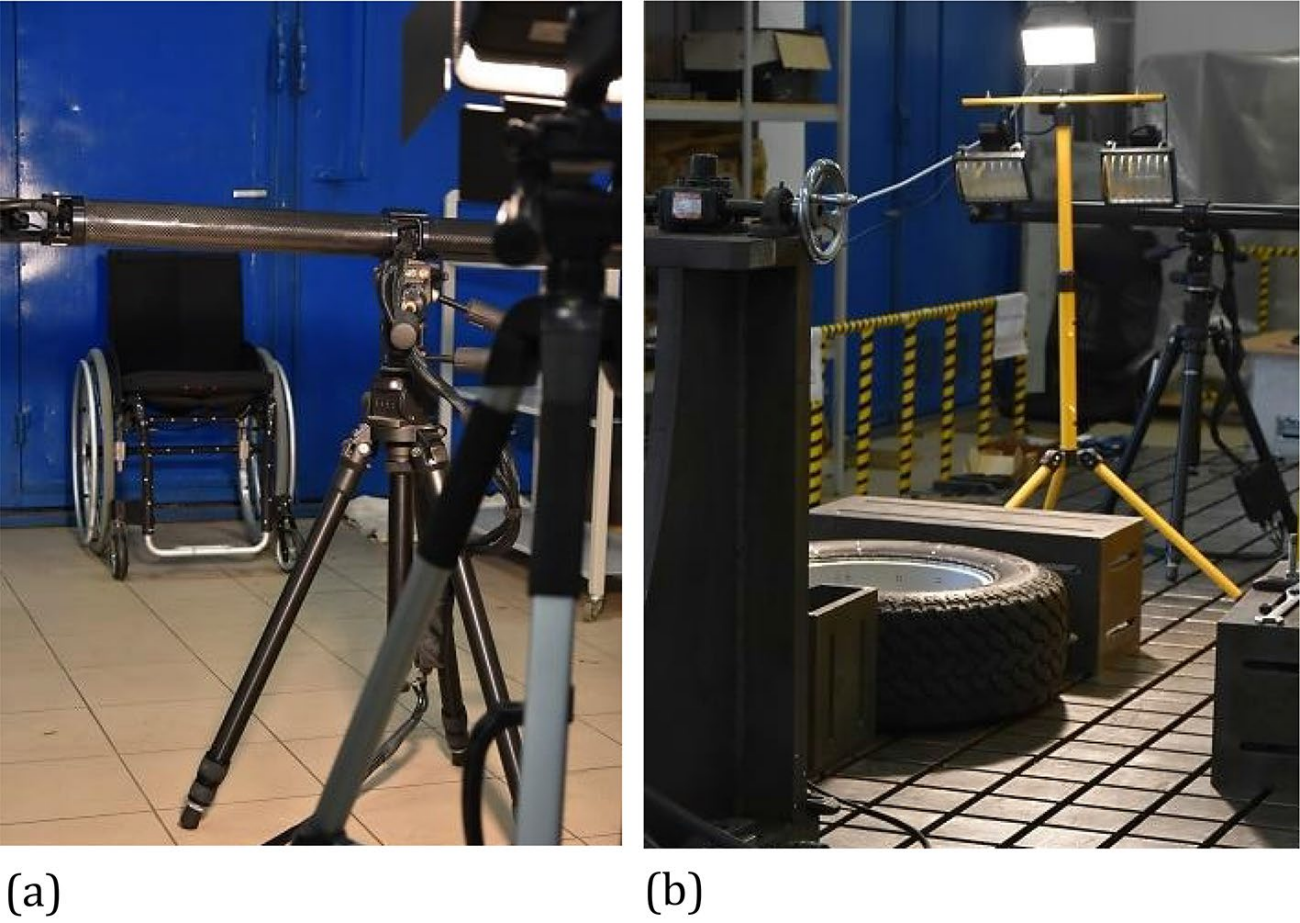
PONTOS 5 M DIC system used in experiments: (a) wheelchair; (b) SUV wheel.
